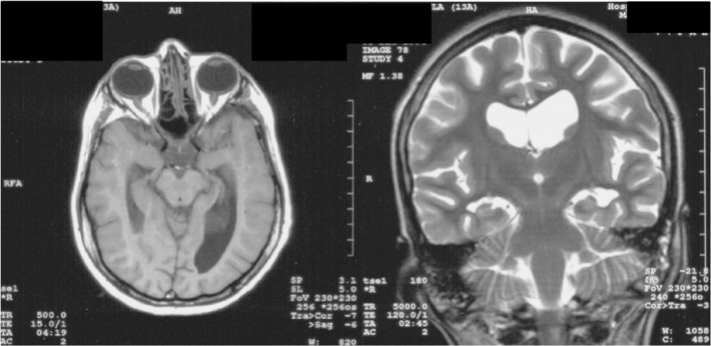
The brain MRI views without contrast at the age of 14 years. They showed cortical dysplasia and asymmetric lateral ventricles with an enlarged right ventricle.
